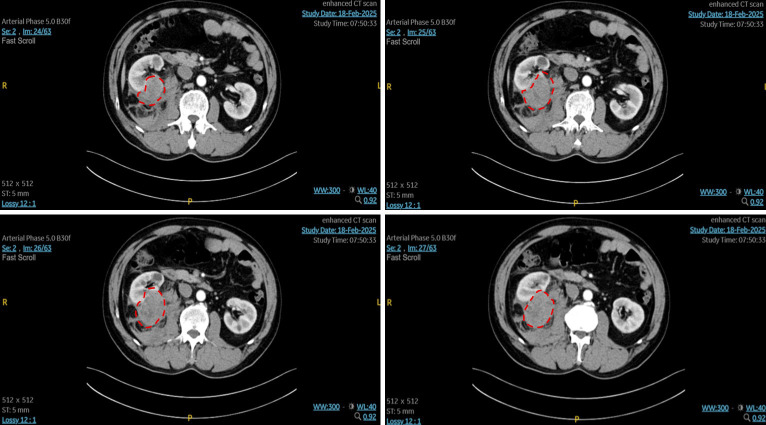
Contrast-enhanced CT (axial view). An ill-defined, heterogeneous mass is seen in the right kidney, measuring approximately 64 × 50 mm, with heterogeneous enhancement on contrast-enhanced imaging. Extensive surrounding patchy and band-like areas of slightly increased attenuation are present, consistent with peritumoral hemorrhage. The perirenal fascia is diffusely thickened, and the interfaces with the duodenum and psoas major muscle are indistinct. Overall, these findings suggest rupture of a right renal tumor with hemorrhage.
